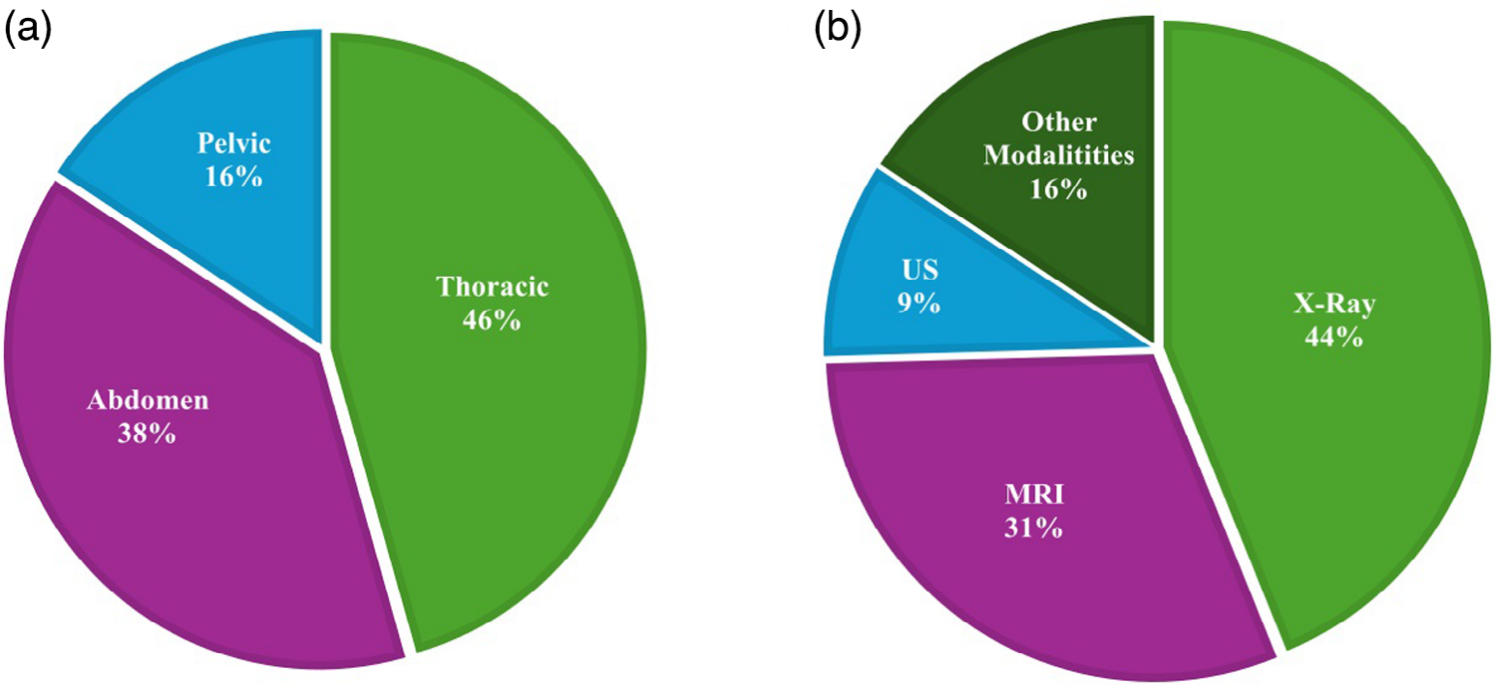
Statistical analysis of artificial intelligence‐based target tracking in PubMed (www.pubmed.gov) since 2005. (a) The percentage of studies conducted on each treatment site. (b) The percentage of each modality in AI‐based motion tracking. X‐ray includes CT, CBCT, 4DCT, MV, and kV fluoroscopic images. Other modalities include respiratory gating, electromagnetic transponders, and optical surface monitoring. (c) The percentage of classic ML versus DL models.
